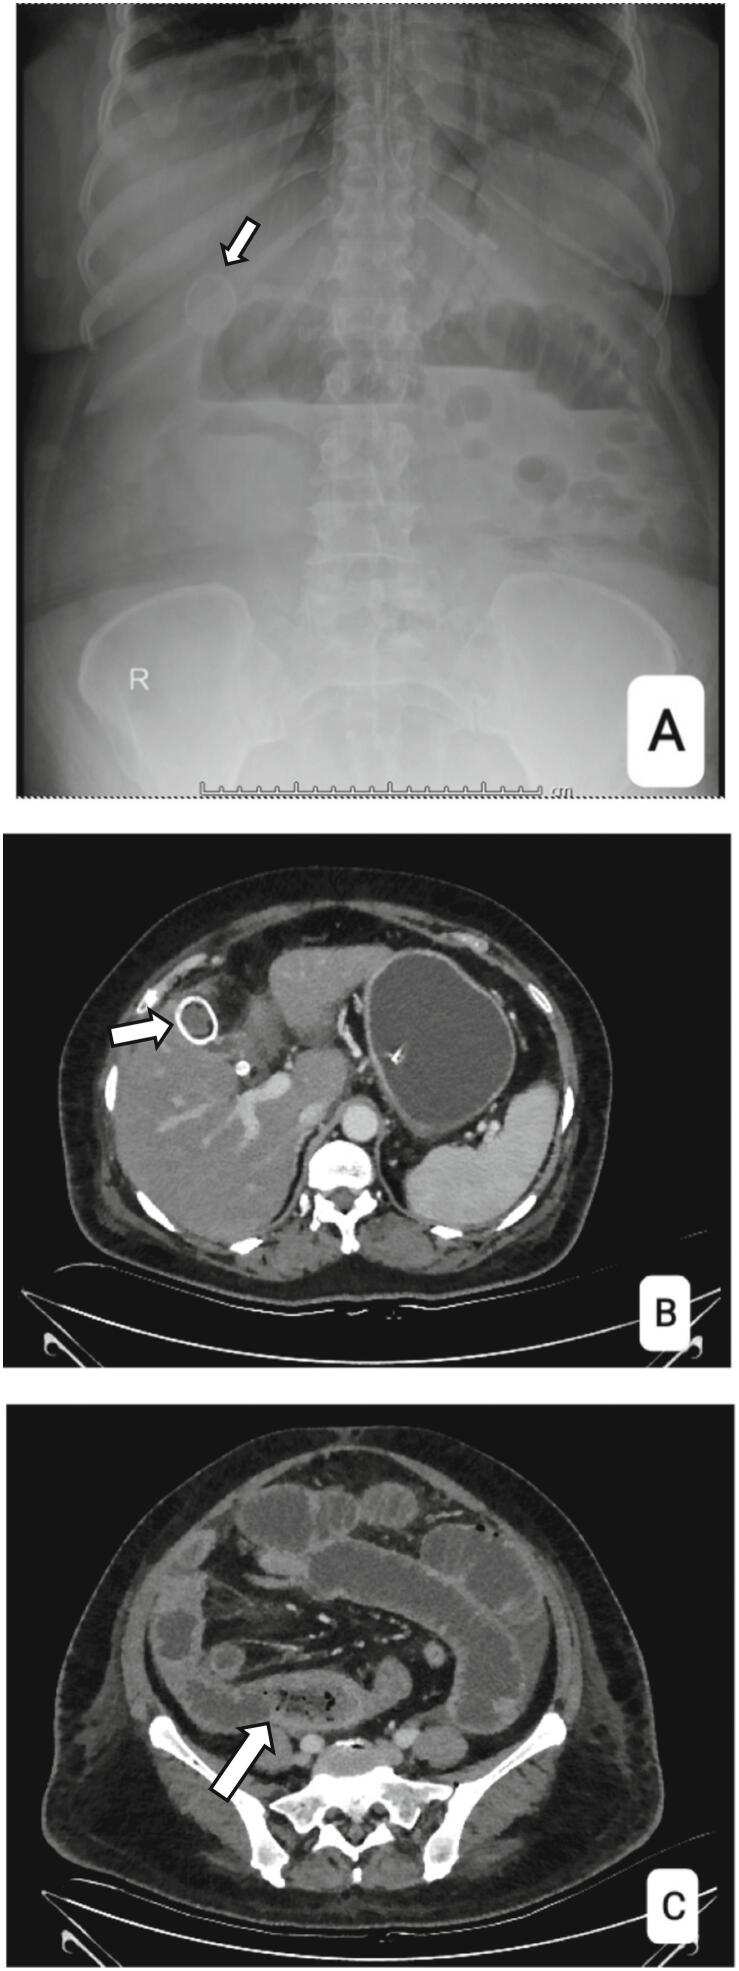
A. X-ray abdomen Erect: Gall bladder calculus and multiple air fluid levels. B. CT Abdomen: Gall bladder calculus. C. Concentric wall thickening with stricture formation in the proximal ileal loops with marked jejunal dilatation. The relevant findings are highlighted by the bold white arrow.
